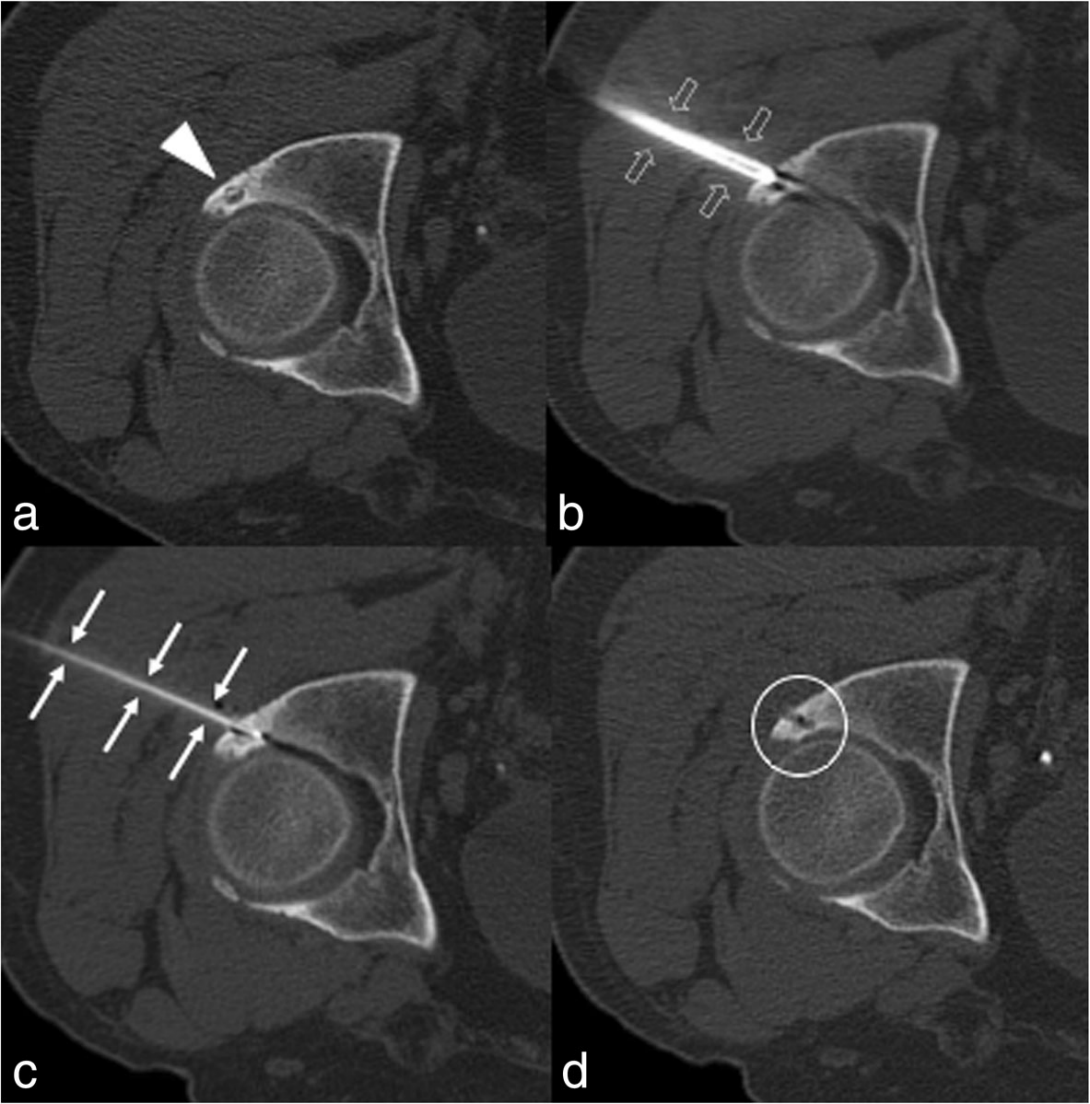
The lesion of the posterior acetabulum (a, headarrow) was biopsied (b, open arrows) and then treated with RFA (c, arrows). Axial CT performed immediately after treatment shows the good results of the ablation (d, circle)
